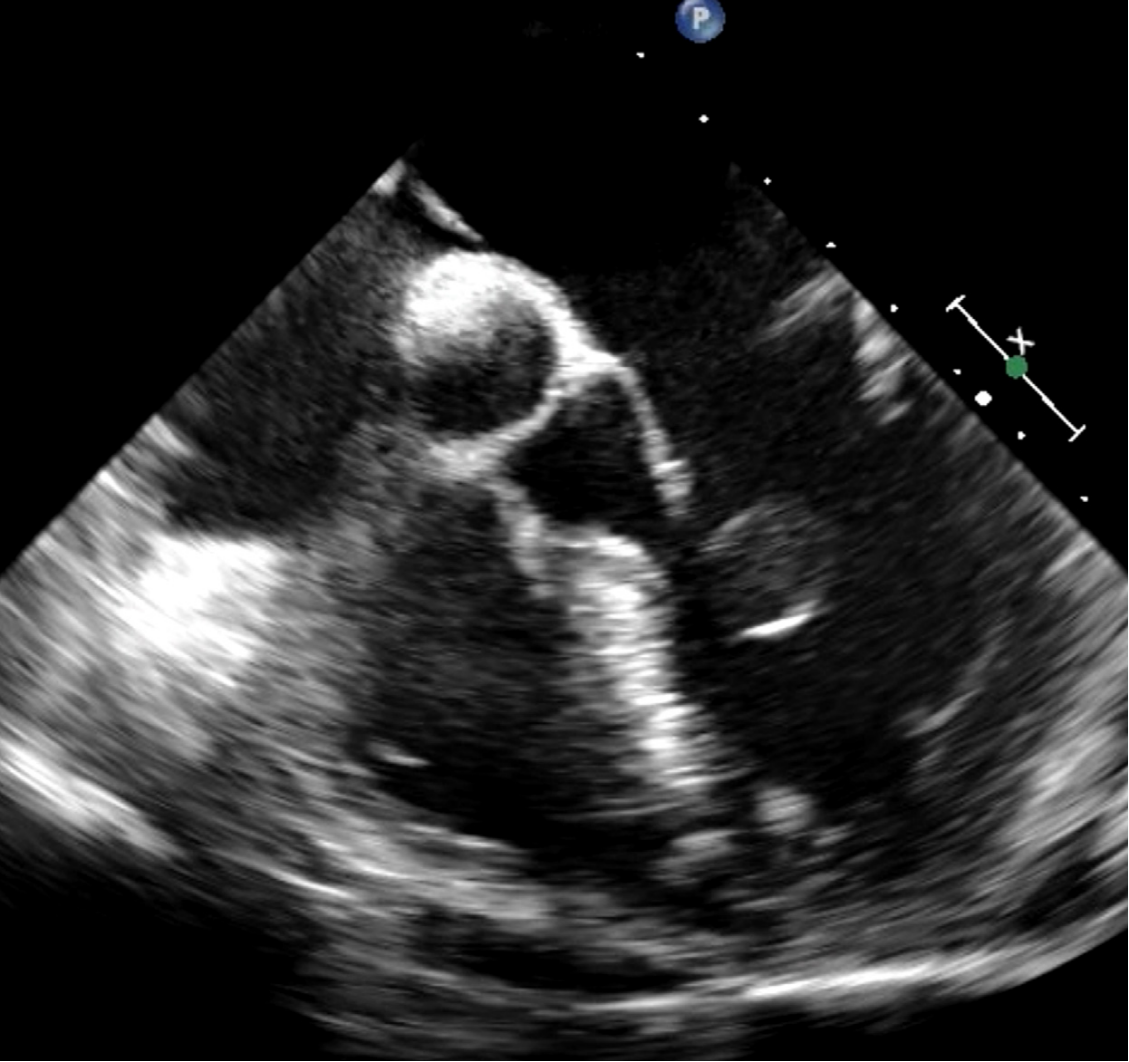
Preoperative transesophageal echocardiography. A 15‐mm cystic round mass was observed in the left ventricle.
